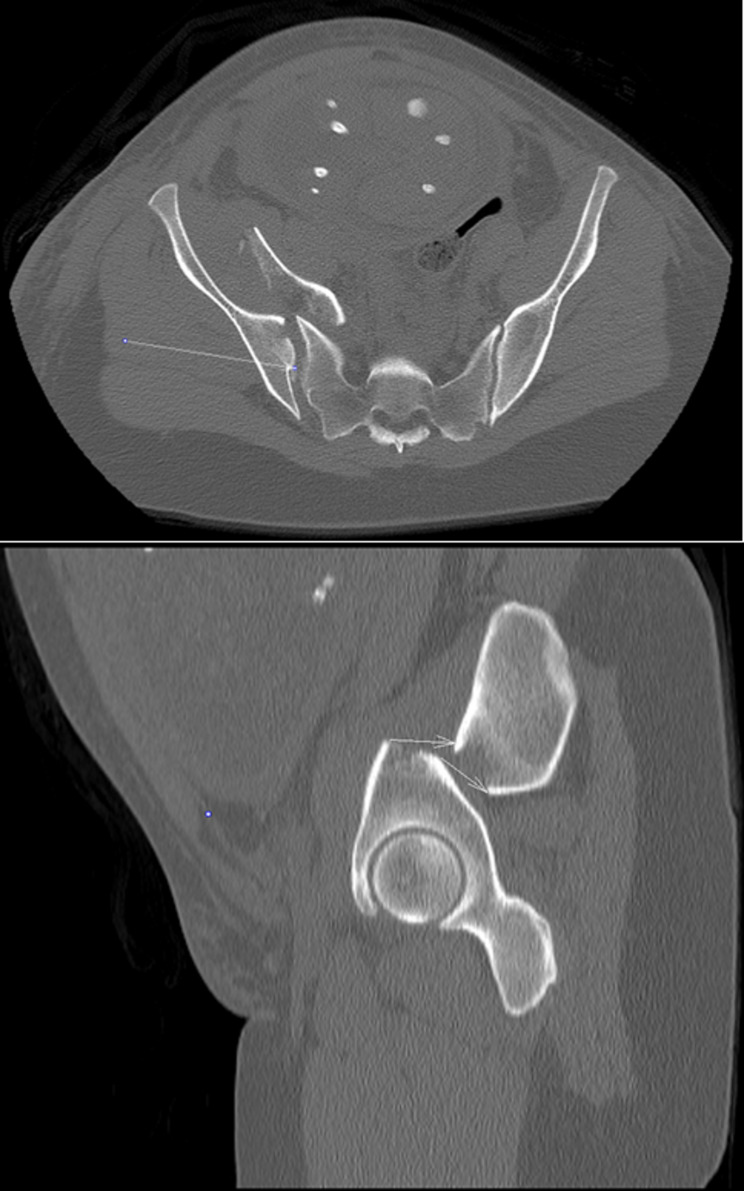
2D CT scan axial and sagittal views represent the fracture of the os ilium with complete bone width dyslocation and sacroiliacal joint dehiscence, anteriorly fetal parts can be seen
